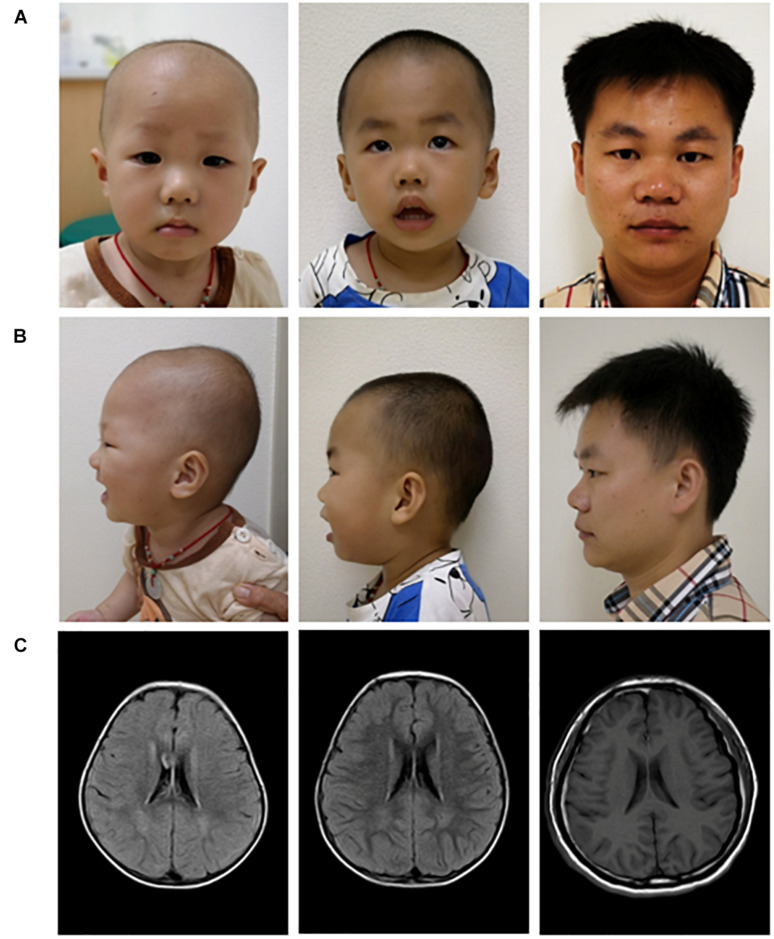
Facial appearance (A,B) and brain MRI images (C) of three carriers with the multi-exon deletion in *PACS1.* Pictures of the proband (left), the elder brother of the proband (middle), and their father (right).
